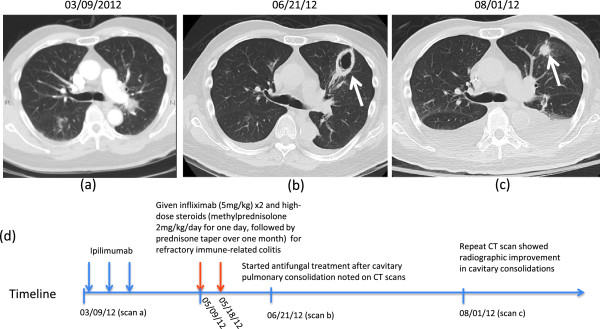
Images and timeline of Aspergillus infection in patient treated with steroids for management of an immune-related adverse event. (a) Baseline chest CT scan prior to ipilimumab. (b) Three weeks after receiving high-dose immunosuppression for immune-related colitis, CT scans showed cavitary pulmonary consolidations (white arrow). Subsequent bronchoalveolar lavage was consistent with *Aspergillus fumigatus* pneumonia. (c) After a 14-day treatment with antifungals, repeat CT scan showed radiographic improvement in cavitary consolidations, but increased bilateral pleural effusions. (d) Timeline of described events (not to scale).
